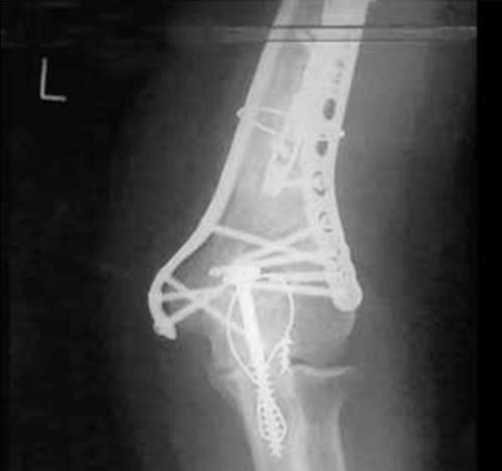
AP radiograph of left elbow post fixation.
